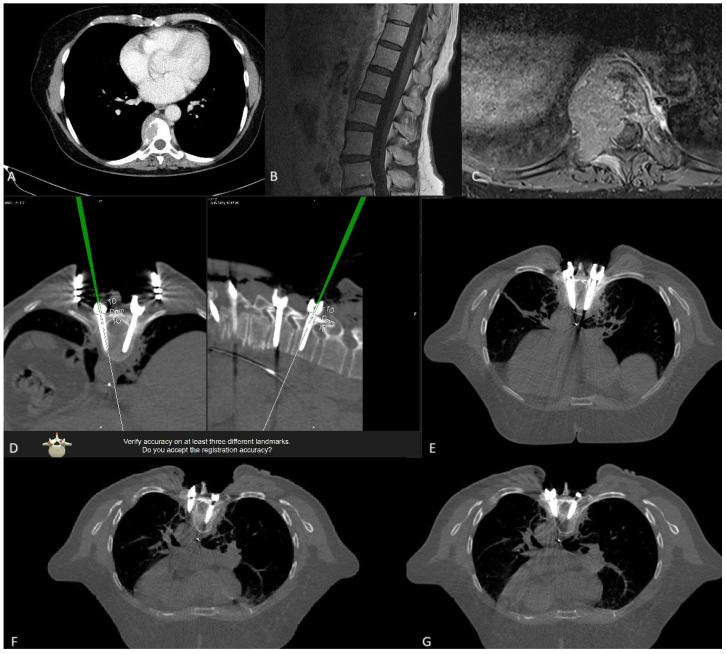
(A) The preoperative CT scan six months before surgery shows an osteolytic lesion in the right Th9 pedicle. A CT-guided biopsy was performed in another department, confirming the tumor, whereupon radiation therapy was initiated. Due to progressive pain with spinal ataxia, an MRI scan of the spine was performed. The preoperative T1 post-contrast (B), sagittal, and (C) axial MRI results show tumor growth from the right pedicle with vertebral body collapse and compression of the dural sac. The patient underwent RG PS placement. The reference array was placed on the spinous process of Th7 and a total of 8 PSs were implanted. (D) Accuracy check with a pointer in the iCT scan to check the PS position. (E). Th8 GRS A PS. (F). iCT shows malposition of the Th7 screw on the left side, a GRS E screw, without any injury to vessels, pleura, or lungs. After removal of the screw, a new RG PS was inserted. (G) The correct position, shown in a repeated control iCT scan.
